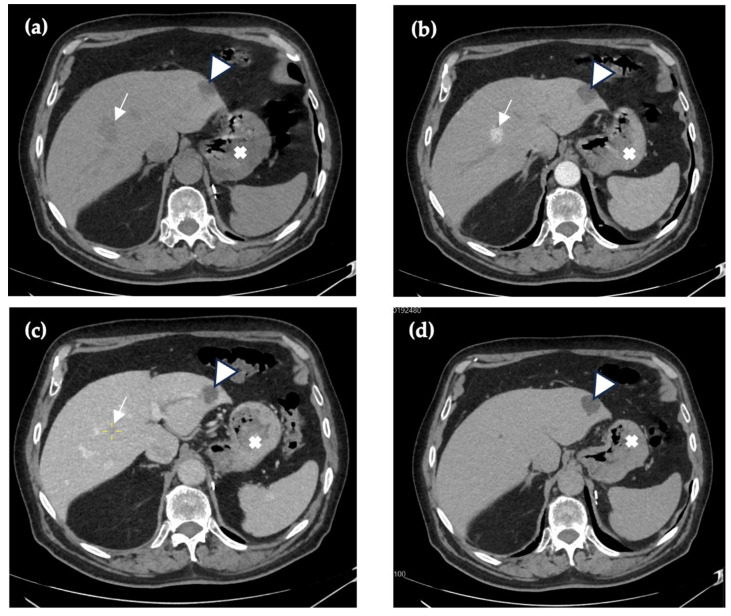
Thirty months after initial imaging, abdominal CE-CT shows a structure (arrow) in the liver with low attenuation consistent with fluid content in native phase (a), there are no infiltrative features or signs of surrounding parenchymal distortion in arterial phase (b) or no appreciable venous (c) enhancement within the lesion, and it merges with liver parenchyma in venous and 5 min delayed (d) phases accordingly. Additionally, a hypoattenuating hepatic cyst in segment II (triangle) and a renal cyst (cross) are visualized consistently throughout all CT phases.
